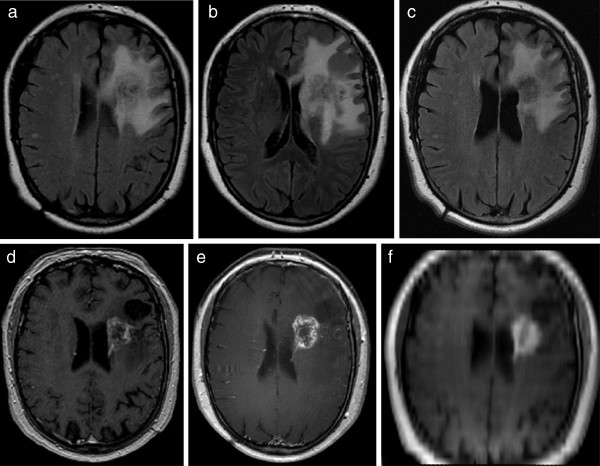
Same patient as Figure 1. Additional follow-up post-treatment examinations reveal constant hyperintensities in FLAIR (a-c) and contrast-enhanced MRI (d-f [f = axial MPR]) six weeks (a,d), three more months (b,e), and 20 months (c,f) after initial post-treatment MRI as shown in Figure 2 indicating non-malignant disease.
